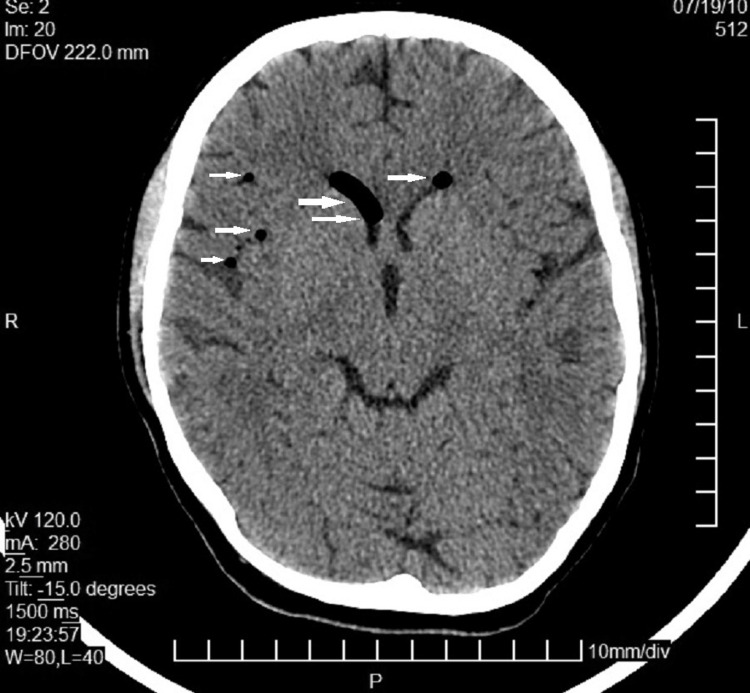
CT day 1 Presence of air intracranially (white arrows)
